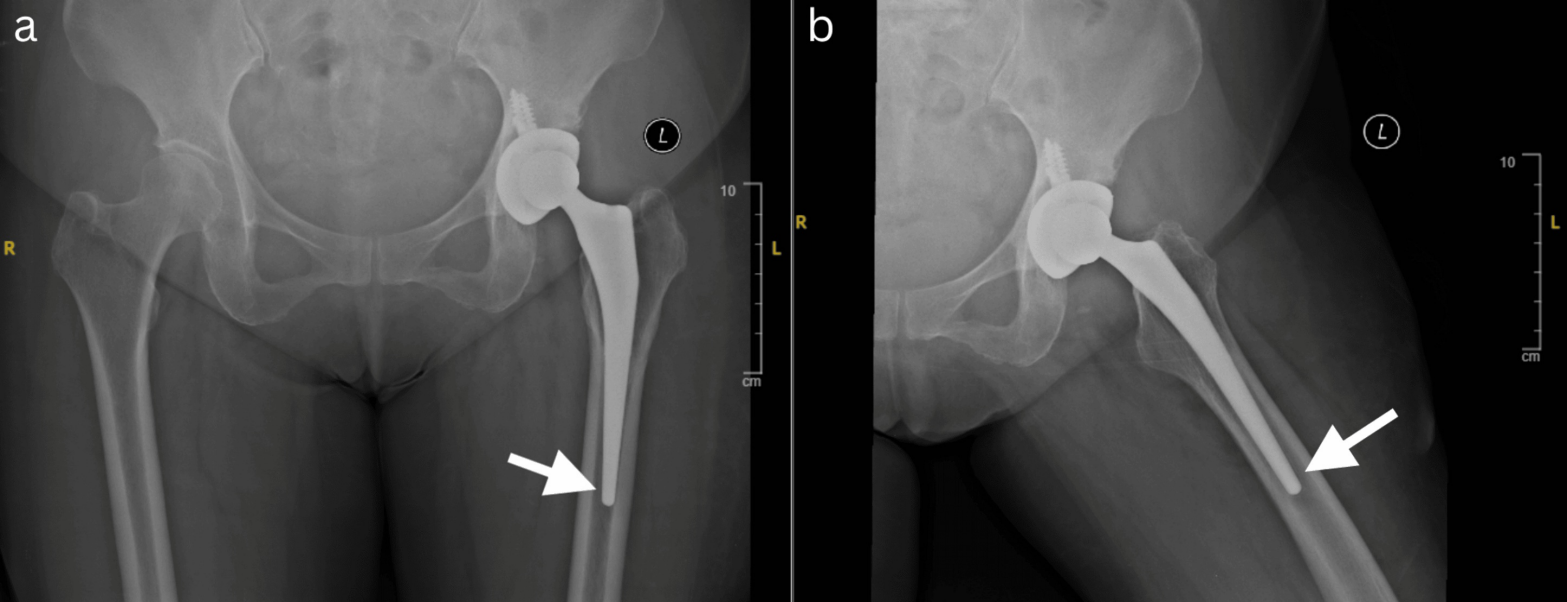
AP (a) and lateral (b) plain radiographs showing radiolucency at the distal stem (see arrow) of the THA
